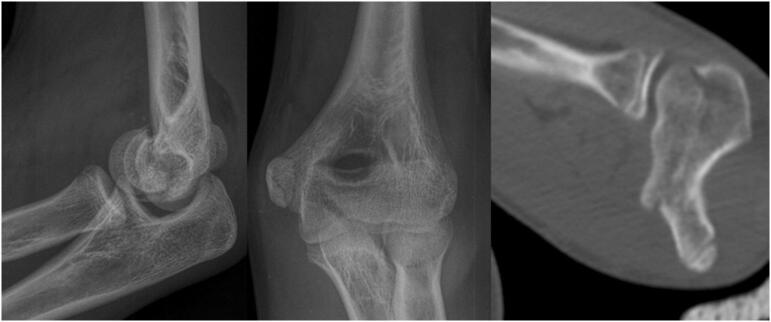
Initial radiographic evaluation of patient 2.
